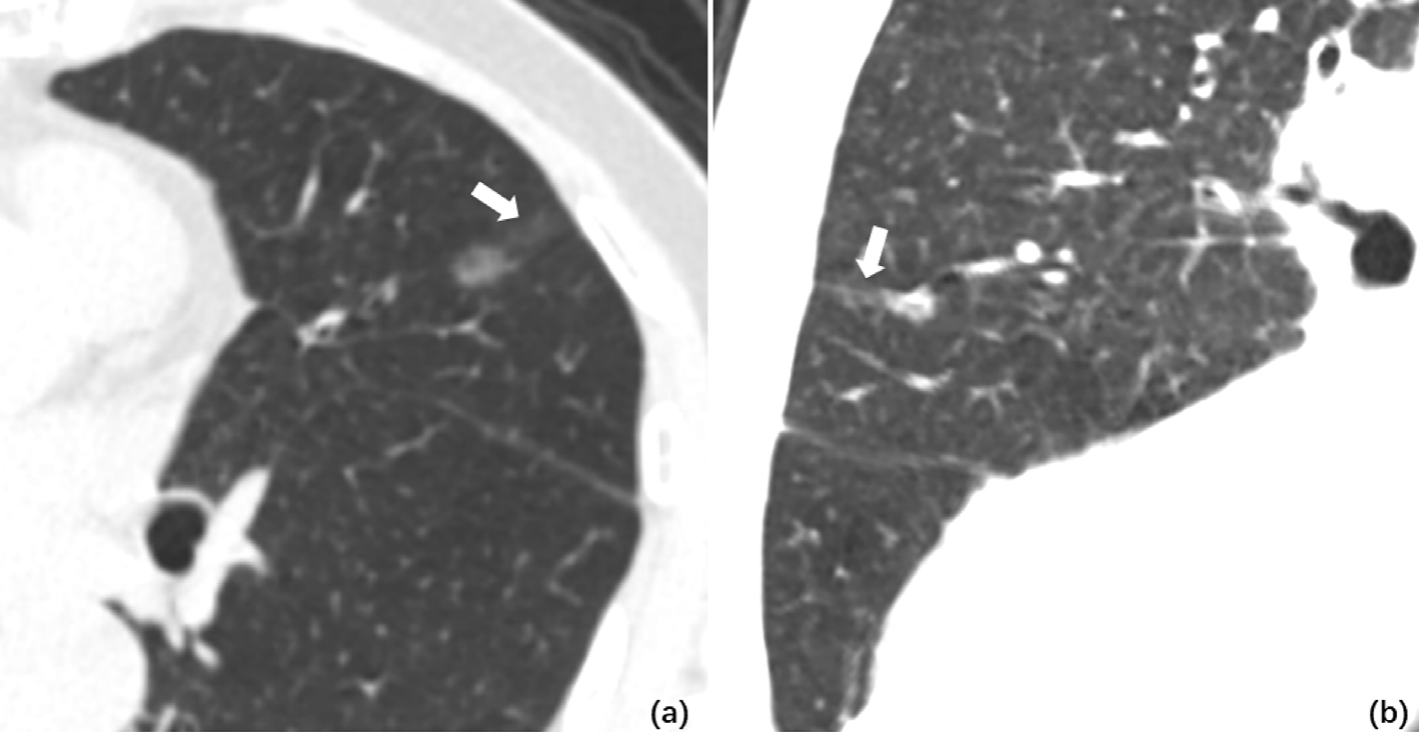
(A) Axial CT image of pGGO performed STAS pathologically. A ground glass ribbon sign was observed on the margin of the lesion and extended to the adjacent costal parietal pleura (white arrow), which was defined as a CT finding of a band-shaped ground glass opacity with blurred edge that emits from the edge of the nodule and extends into the adjacent lung (B). Multi-plane reconstruction with ground glass ribbon sign as the long axis. The shape of ribbon sign was displayed more clearly after adjusting the window width and level.
